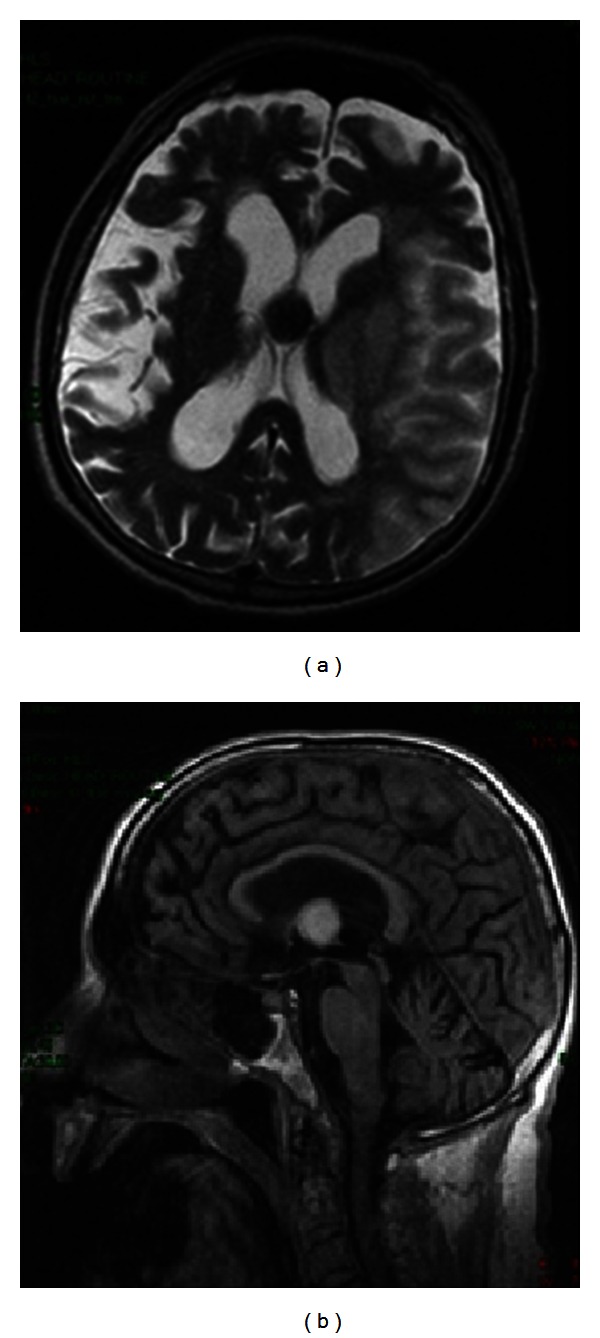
Colloid cyst. Round, well-demarcated lesion of third ventricle, hypointense on T2-weighted (a) and hyperintense on T1-weighted (b) MR images. Department of Neurology, Colentina Clinical Hospital brain imaging archive.
